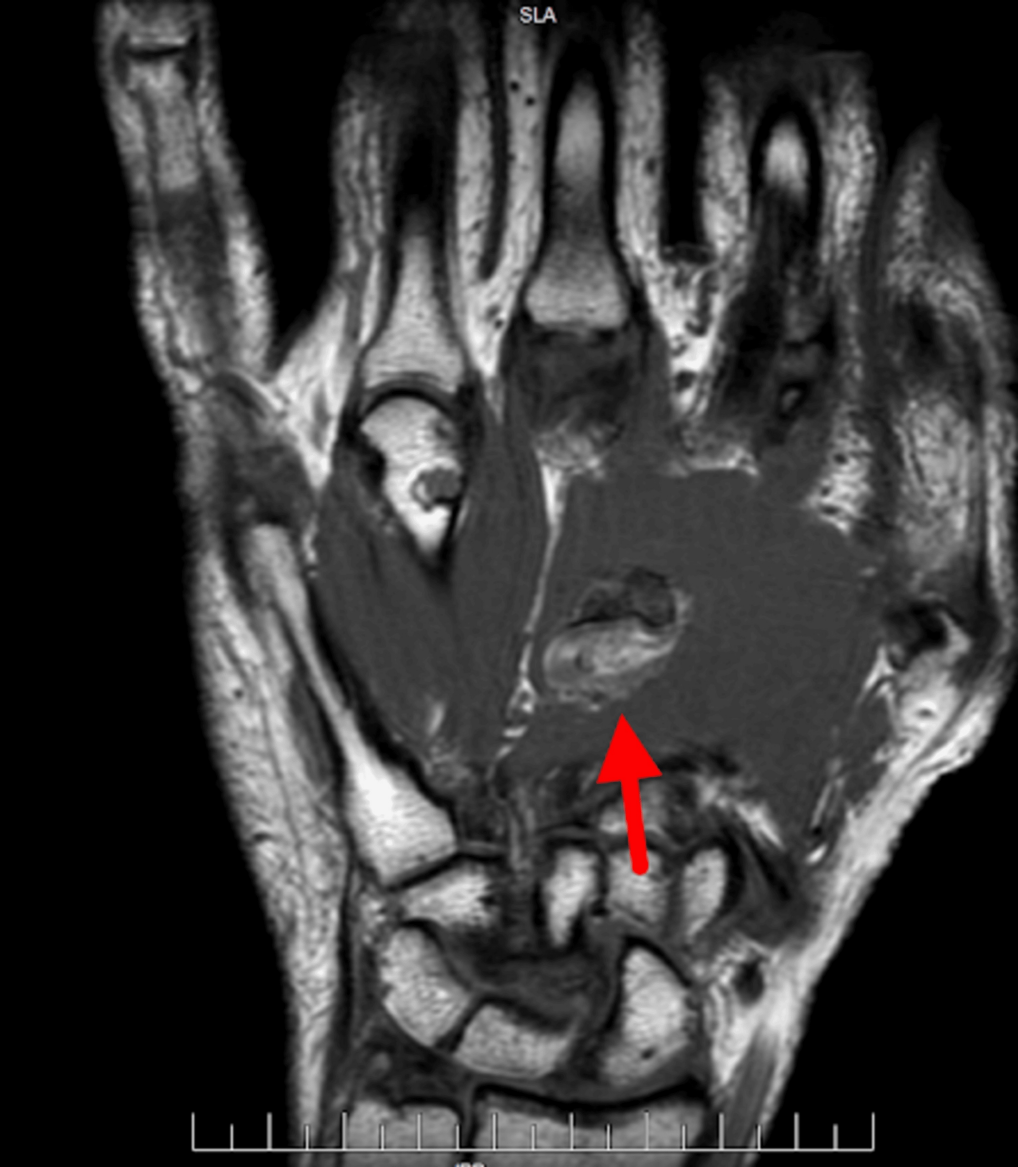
MRI imaging Demonstrates a 1.9 × 1.8 × 1.2 cm heterogeneously enhancing ovoid soft-tissue mass within the palmar tissues, centered over the proximal shaft of the third metacarpal and extending into the interspace between the second and the third metacarpals.
